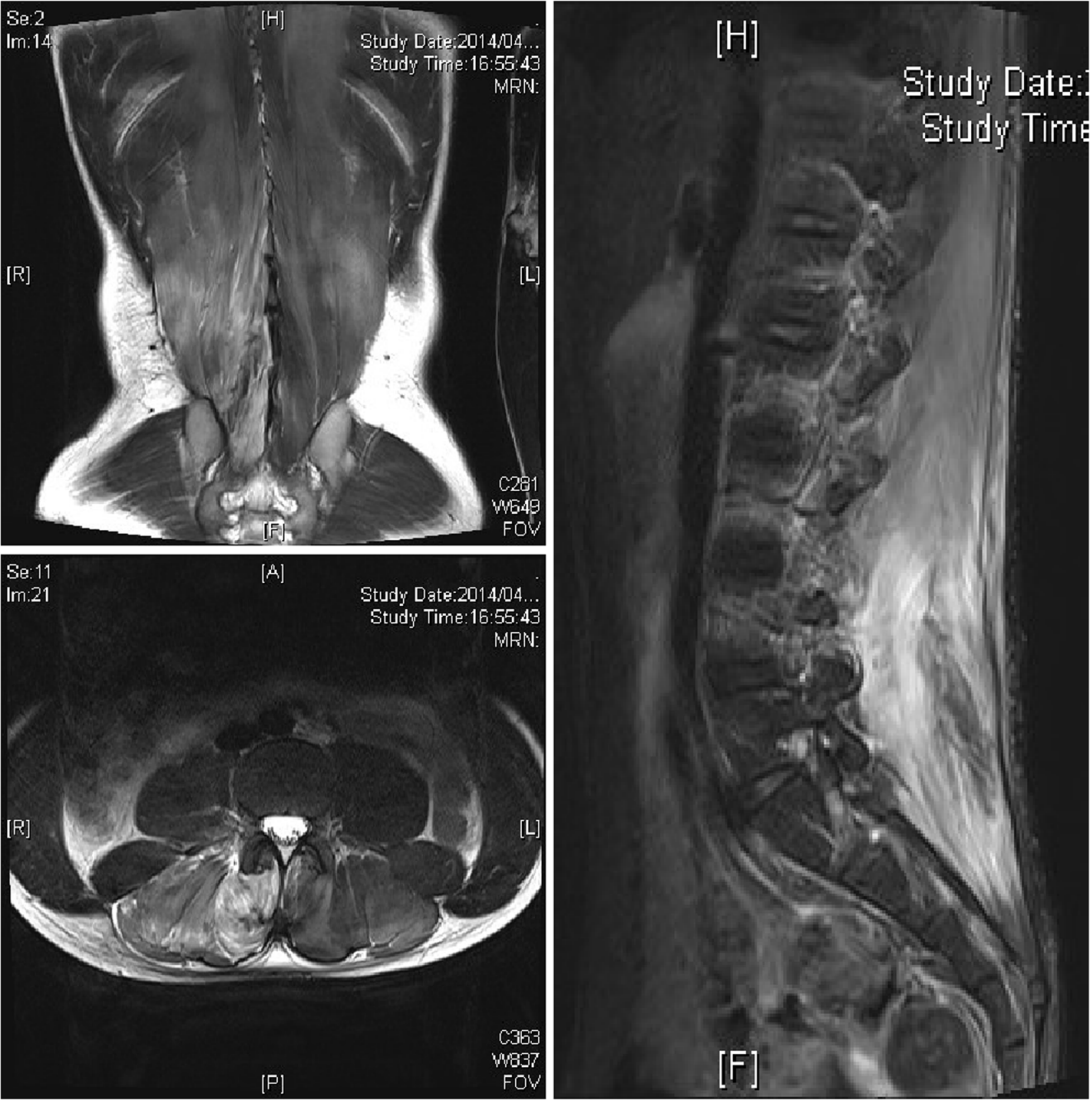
Magnetic resonance imaging after 7 days. Decrease in edema was seen on T2-weighted images
